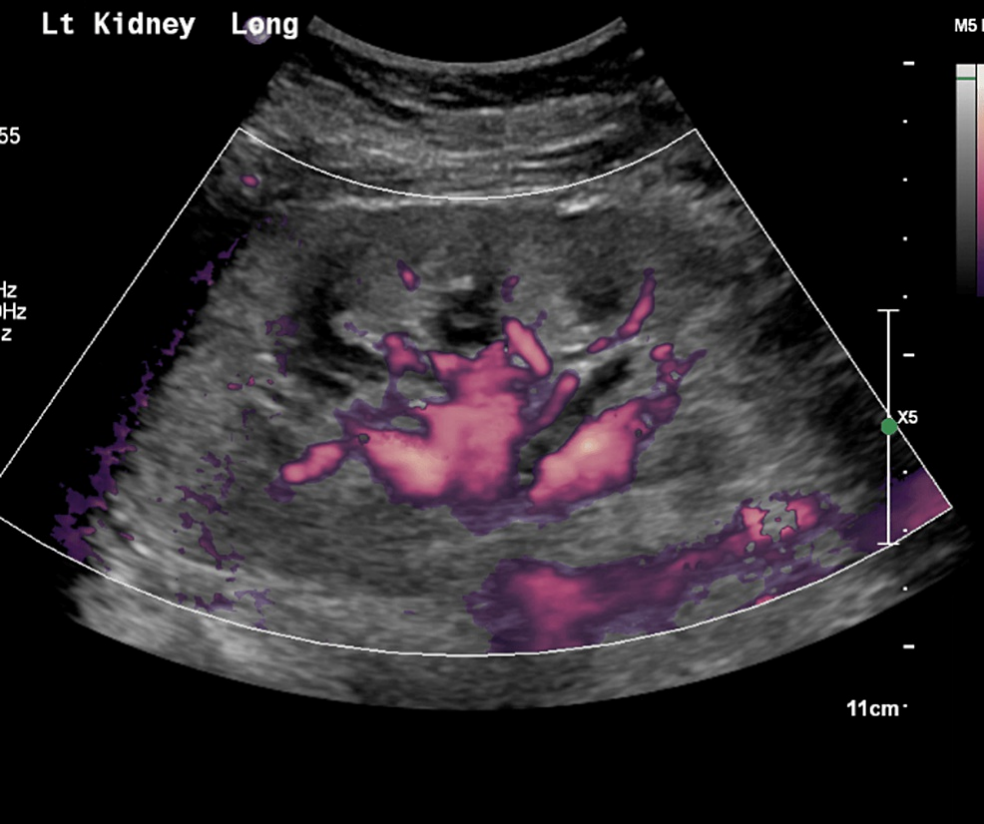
Doppler ultrasound of left kidney: Increased renal echogenicity, consistent with medical renal disease.
